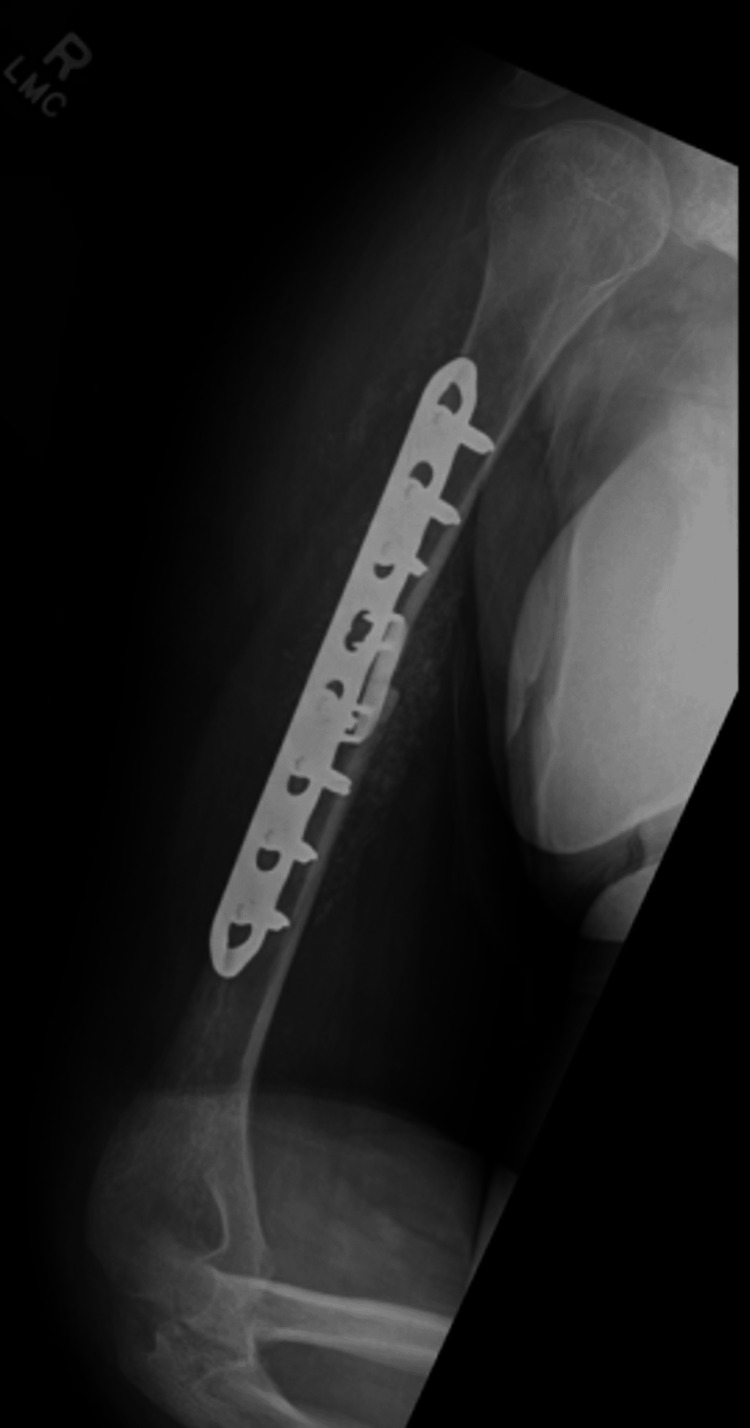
Immediate post-operative radiograph of the right humerus. Eight-hole plate and nitinol staple noted bridging the fracture site.
